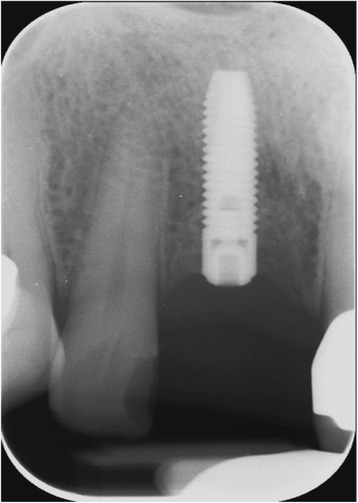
Single tooth X-ray, showing a constant bone level 7 months after implant placement
